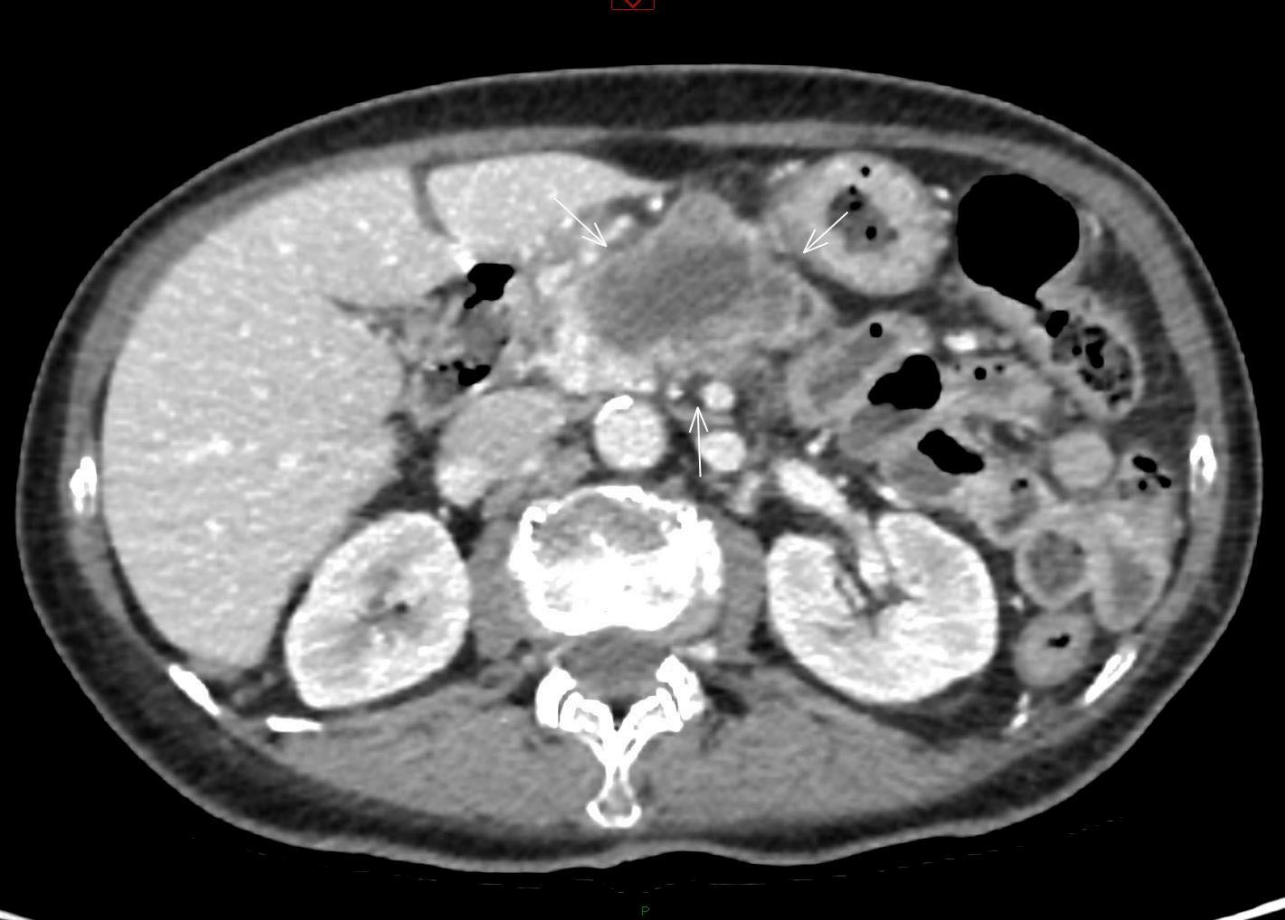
Radiologic progression of pancreatic metastasis after second-line chemotherapy. Contrast-enhanced CT shows a hypodense, irregular, heterogeneous mass in the pancreatic body junction, measuring 5.2 × 4.4 cm, with distal pancreatic atrophy and main pancreatic duct dilatation. The lesion invades the splenic and superior mesenteric veins, demonstrates approximately 180° circumferential contact with the splenic artery, and abuts the left hepatic lobe.
